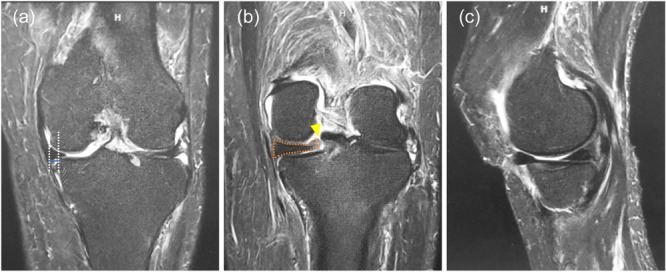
Patient characteristics and imaging findings of a female patient cohort diagnosed with MMPRT on MRI. (a) MME (blue line) is observed. No bone marrow lesion is detected in the MFC and MTP (coronal images). (b) Giraffe neck sign (orange dotted line) and cleft sign (yellow arrowhead) are observed. (c) No bone marrow lesion is observed in the medial compartment (sagittal images). MFC, medial femoral condyle; MME, medial meniscus extrusion; MMPRT, medial meniscus posterior root tears; MRI, magnetic resonance imaging; MTP, medial tibial plateau; SIFK, subchondral insufficiency fractures of the knee.
